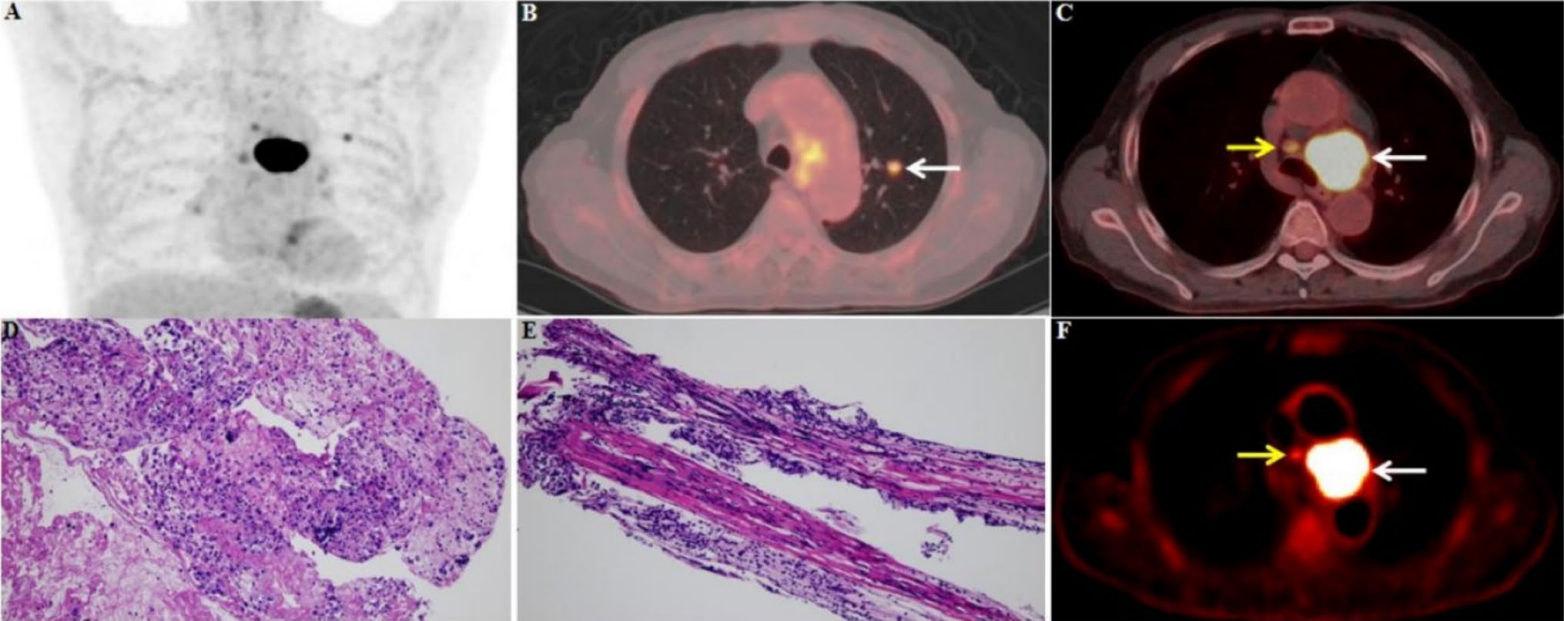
dPET/CT and sPET/CT images of non-metastatic and metastatic FDG-avid LNs
